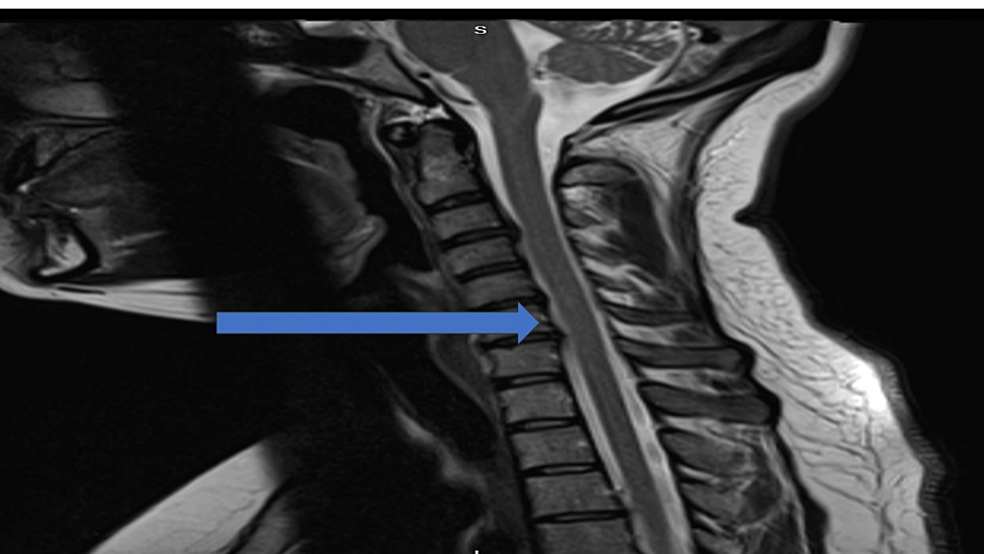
Sagittal view of the cervical spine on magnetic resonance imaging for the patient Above is a sagittal cut from the cervical spine MRI of the patient in the case report. The area marked with the arrow demonstrates the area where the patient nerve roots for the affected area would disperse from. The view also shows that there is no significant compression at this level which could cause the patient's symptoms.
